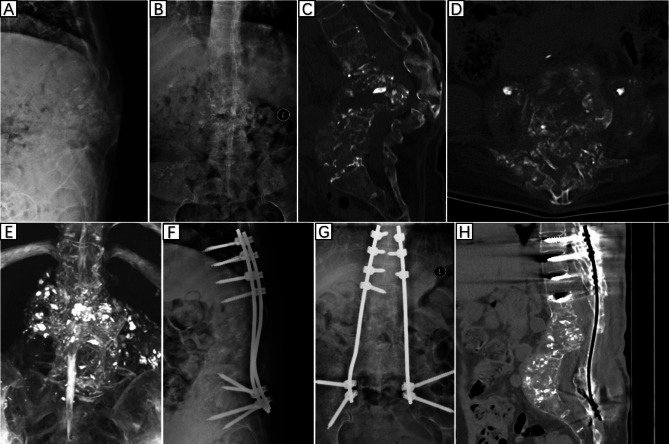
(A and B) Preoperative X-ray examination. (C-E) Preoperative CT examination. (F and G) Postoperative X-ray examination. (H) Postoperative CT examination
